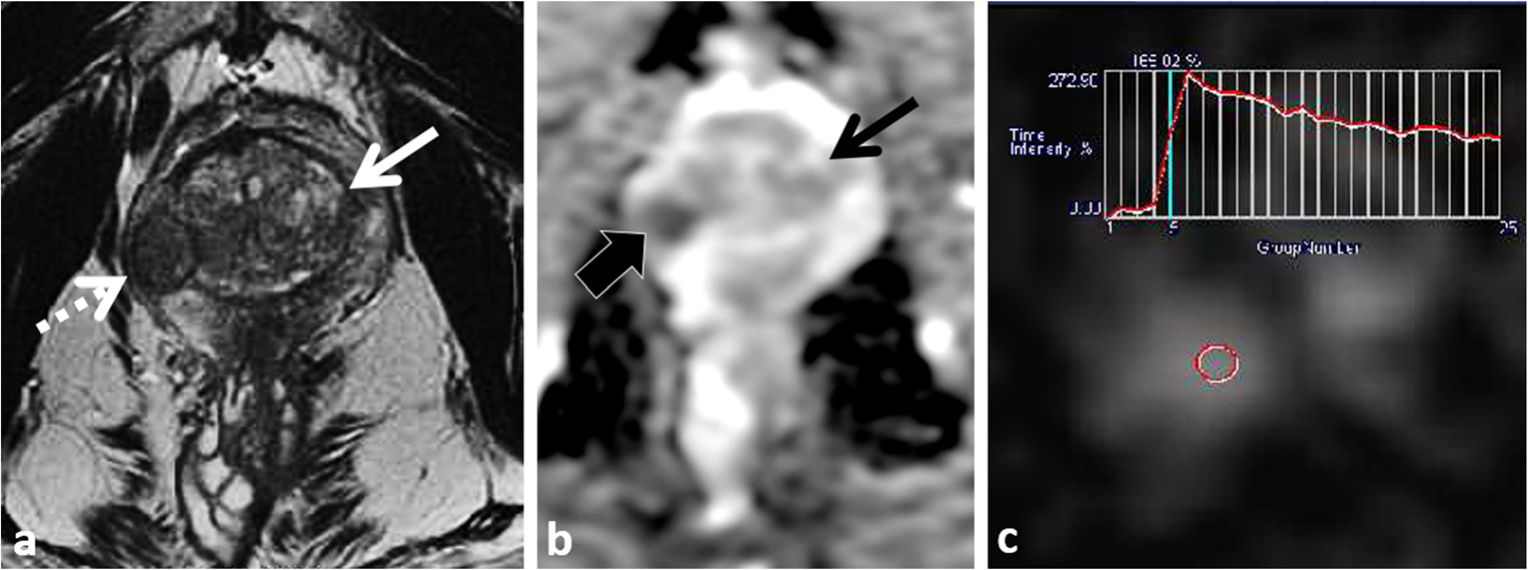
A 47-year-old patient post-total colectomy for ulcerative colitis and elevated PSA. MP-MRI was performed to evaluate for potential tumour and to plan a biopsy. Axial T2W TSE (a) image demonstrates enlargement of the central gland (TZ) consistent with BPH. There is a well-circumscribed, round, mixed but predominantly increased T2W SI nodule with internal cystic change (*white arrow*) and a predominantly homogeneously low T2 SI nodule at the junction of the right middle PZ and central gland (*dotted arrow*). The larger nodule demonstrates T2 shine-through on the ADC map (*black arrow* in b) and is characteristic of glandular BPH. The other nodule demonstrates restricted diffusion (*thick black arrow* in b) and type III kinetics at DCE (c). A diagnosis of prostate cancer was suggested. Targeted biopsy revealed normal prostatic tissue and stromal BPH. Follow-up MRI (not shown) demonstrated no change and the PSA was stable. In retrospect, the nodule is round, well-circumscribed and demonstrates a complete low T2 SI rim (a), findings that are more in keeping with stromal BPH rather than cancer
